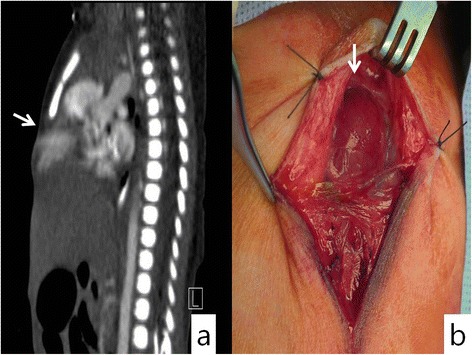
Chest computed tomography (CT) of an infant with cardiac defect showing (a) protrusion of the cardiac apex through dehiscent chest wall and no ossification center at the lower sternum and (b) intra-operative findings showing four defects in the lower sternum, abdominal wall, anterior portion of the diaphragm, and diaphragmatic portion of the pericardium.
